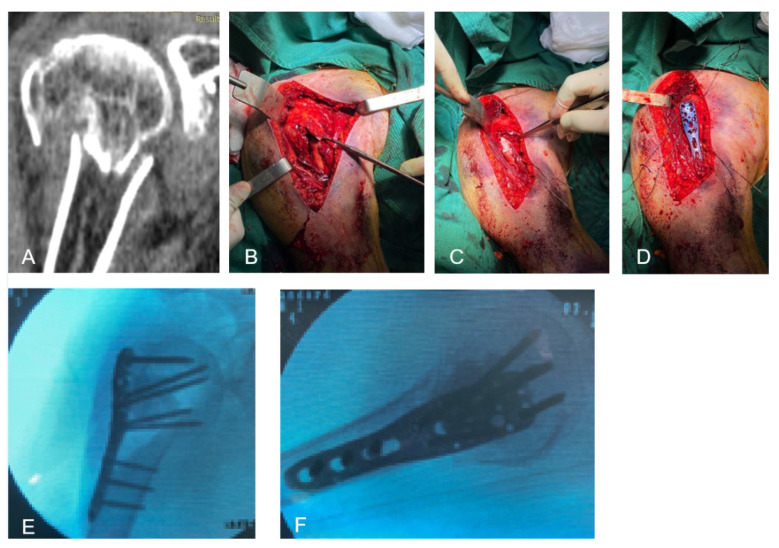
(A) Preoperative computed tomography scan with bone impaction and metaphyseal void; (B,C) intraoperative images with metaphyseal void and PMMA cement placement through the lateral window; (D) plate positioning; (E,F) anteroposterior and lateral intraoperative X-ray images.
